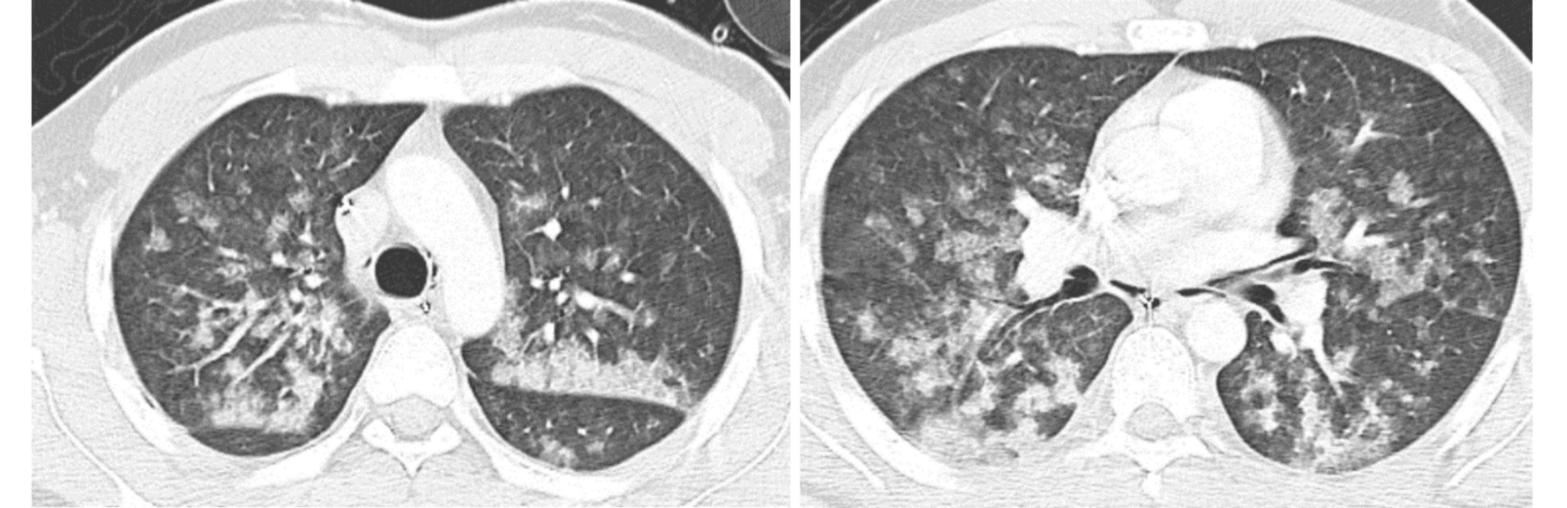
CT scan of the thorax showing alveolar hemorrhage
